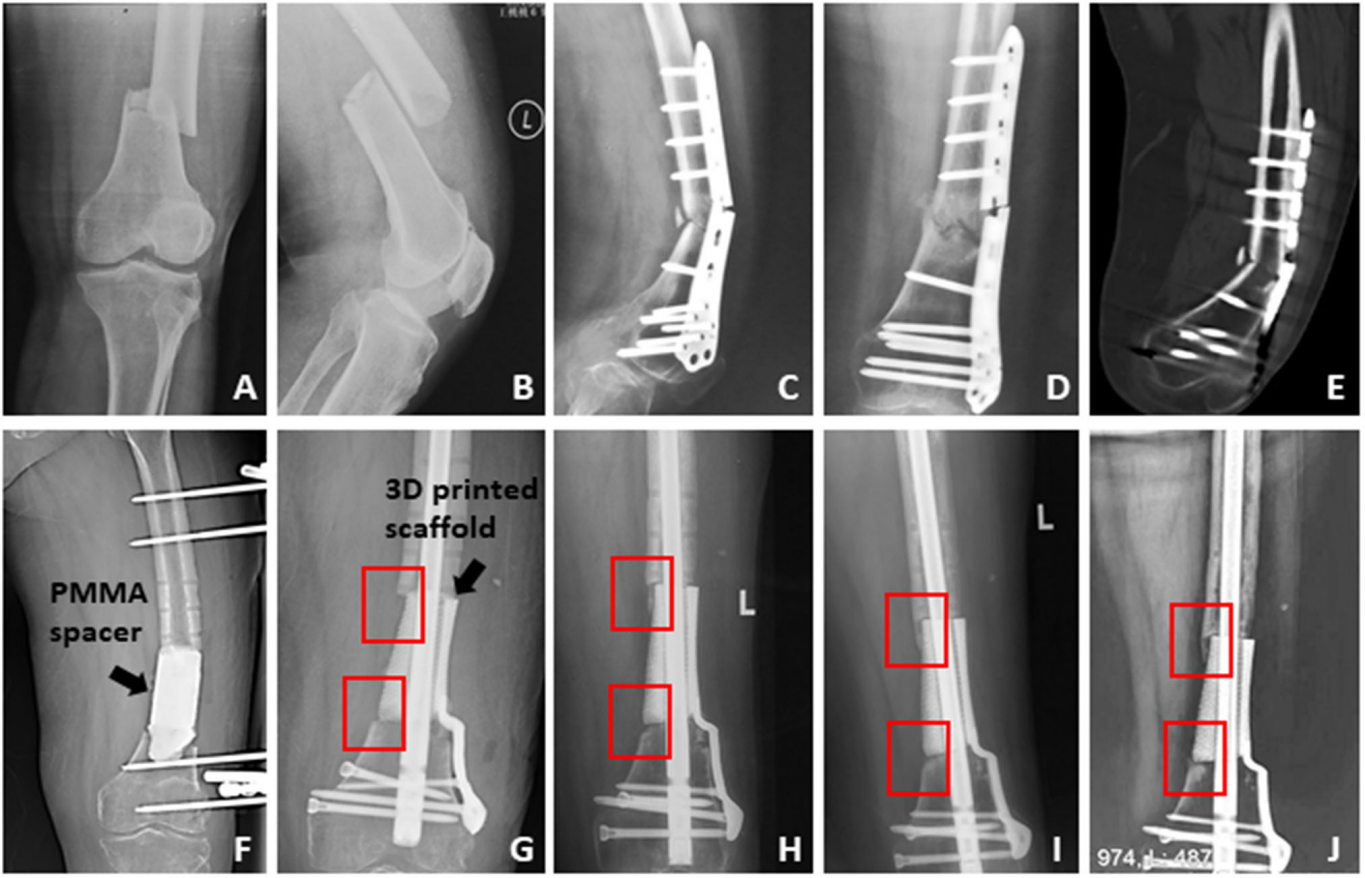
The therapeutic process of the first typical case. A, B The initial X-ray displayed the patient suffered the distal femoral displaced fracture; C–E The patient underwent the surgical treatment, and at 13 months after the initial surgery, X-ray and CT scan showed fracture nonunion and ends sclerosis, meanwhile, the plate had broken; F we cleared the sequestrums and filled a PMMA spacer into the femoral defect with 9.5 cm length, and stabilized the femur by external fixator; G–J after a 10-week interval, the bone defect was repaired by a customized 3D printed scaffold, and the postoperative regular X-rays showed the scaffold kept stable and new bone grew gradually on the surface of scaffold from both ends of the defect (as displayed in the red boxes)
